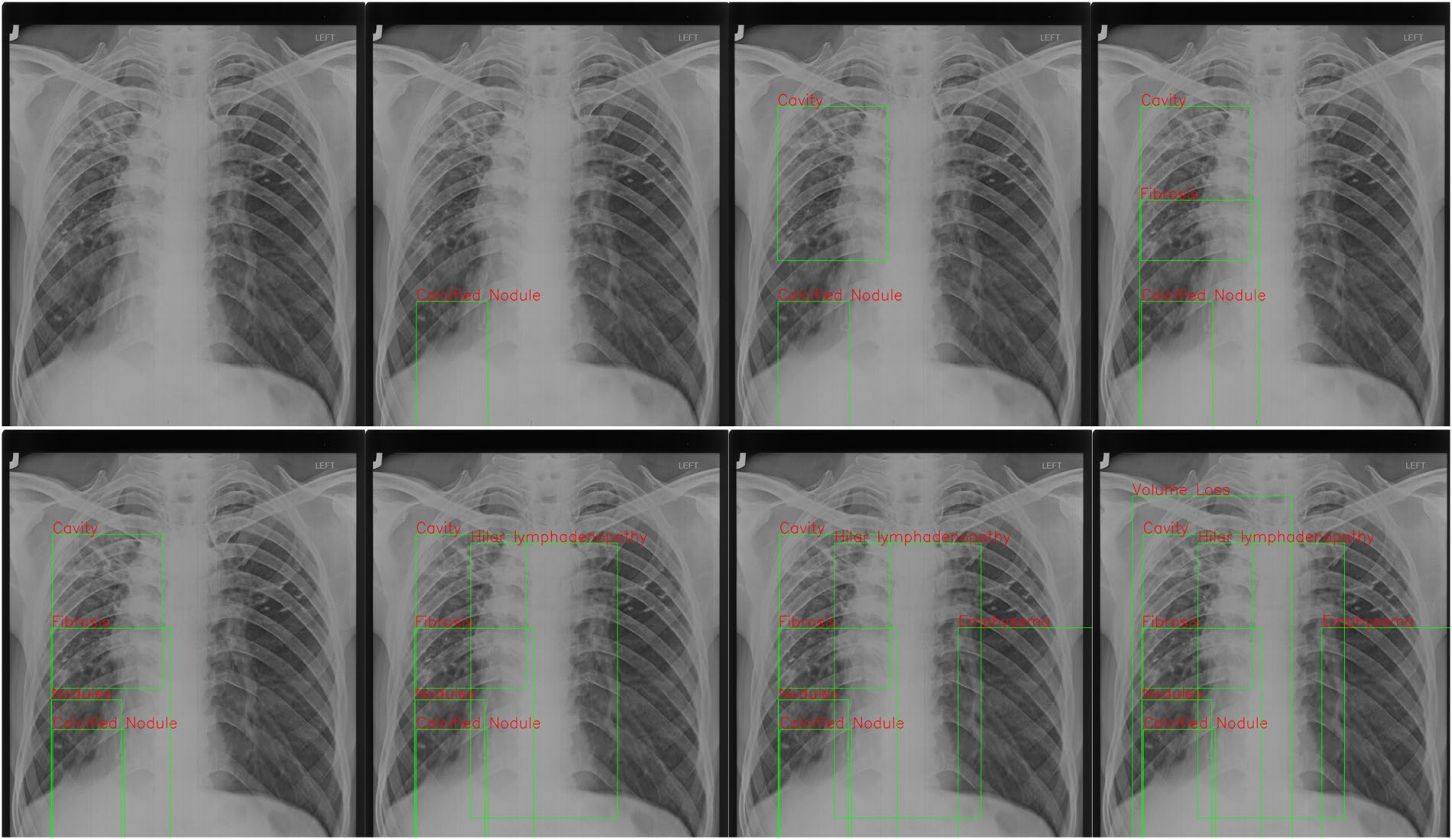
Showcasing the annotations for different findings for a given chest X-ray image. Each finding is shown as a bounding box and effectively highlights the annotation methodology and provides valuable insight into the range of findings that can possibly exist in the Silicosis and related disorder-affected Chest X-rays. The Figure showcases the progression of each finding to be clearly visualized without interference from other annotations and effectively demonstrates how each annotation appears on the image, providing a clearer understanding of the localization of different findings.(Best viewed in colour).
